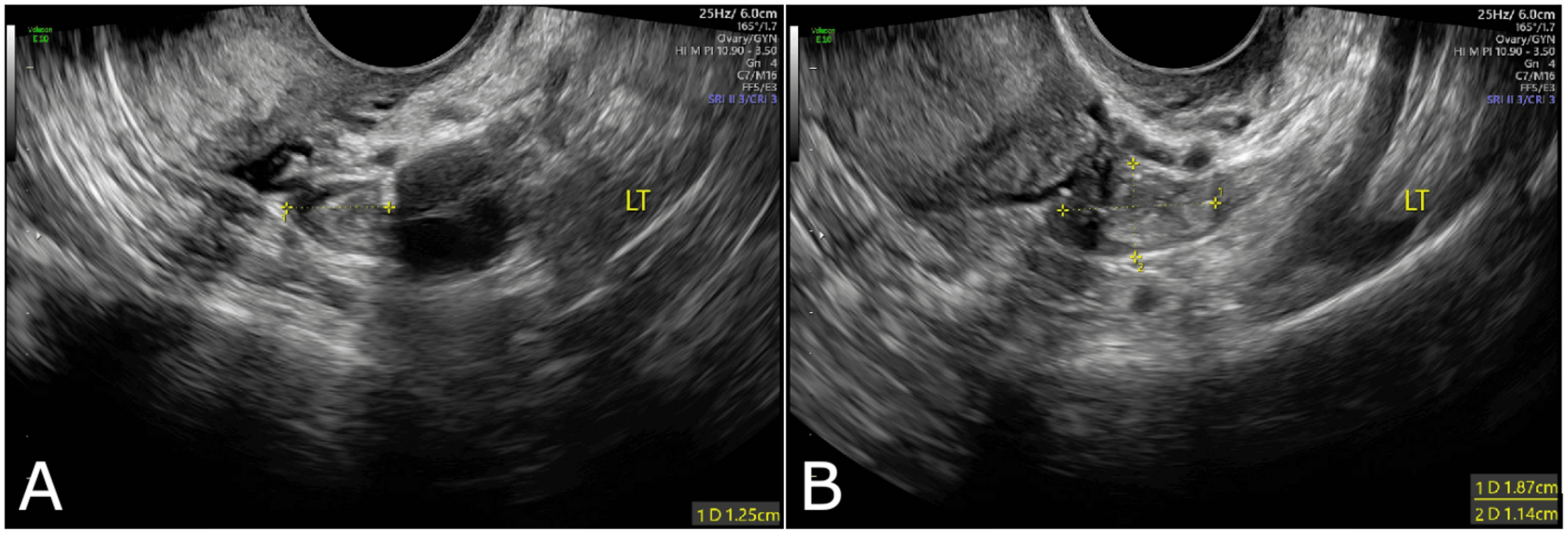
Pelvic Ultrasound Pelvic ultrasound demonstrating a hemorrhagic structure adjacent to the left ovary measuring 1.25 x 1.87 x 1.14 cm.
